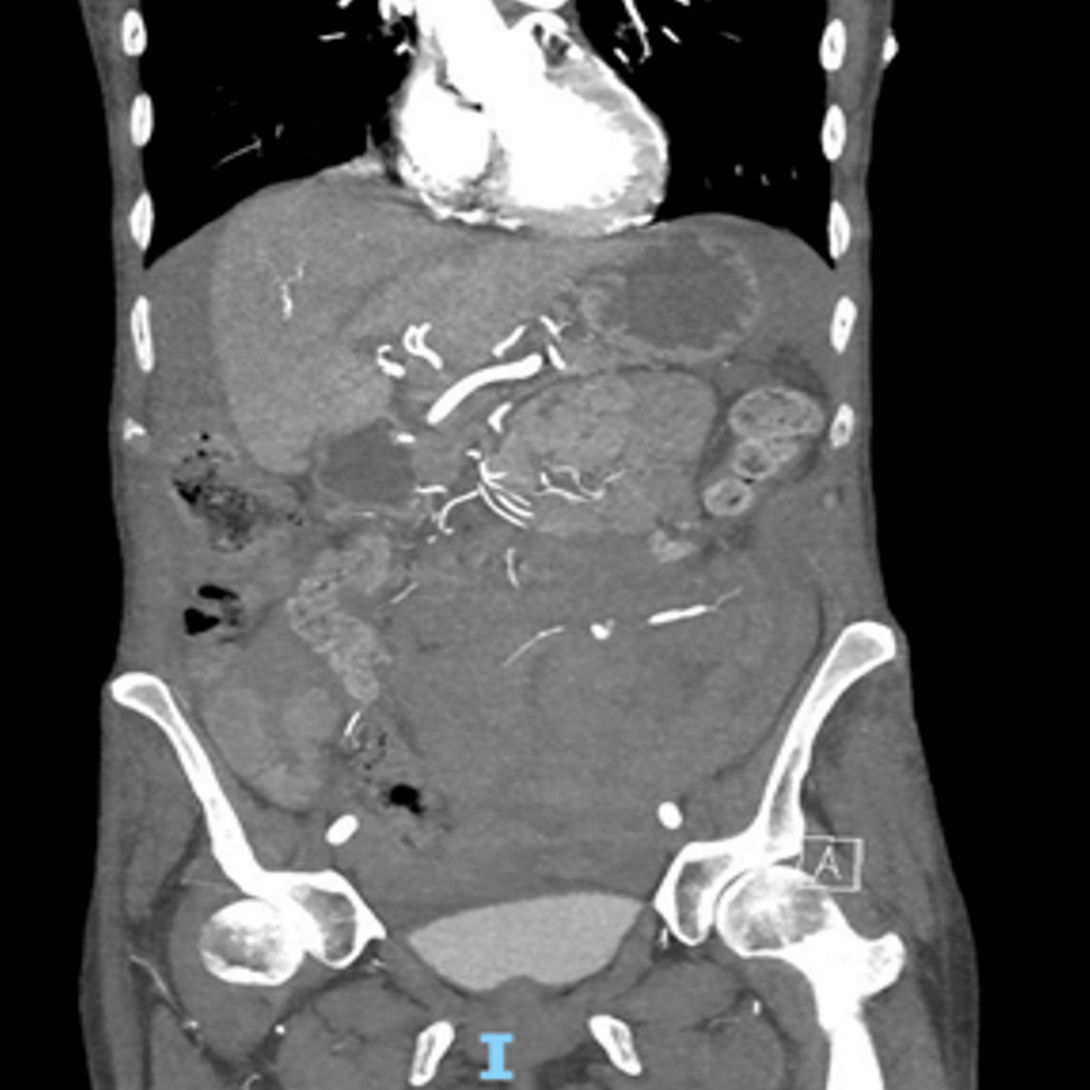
Arterial phase CT - coronal view showing active arterial blush CT: computed tomography
